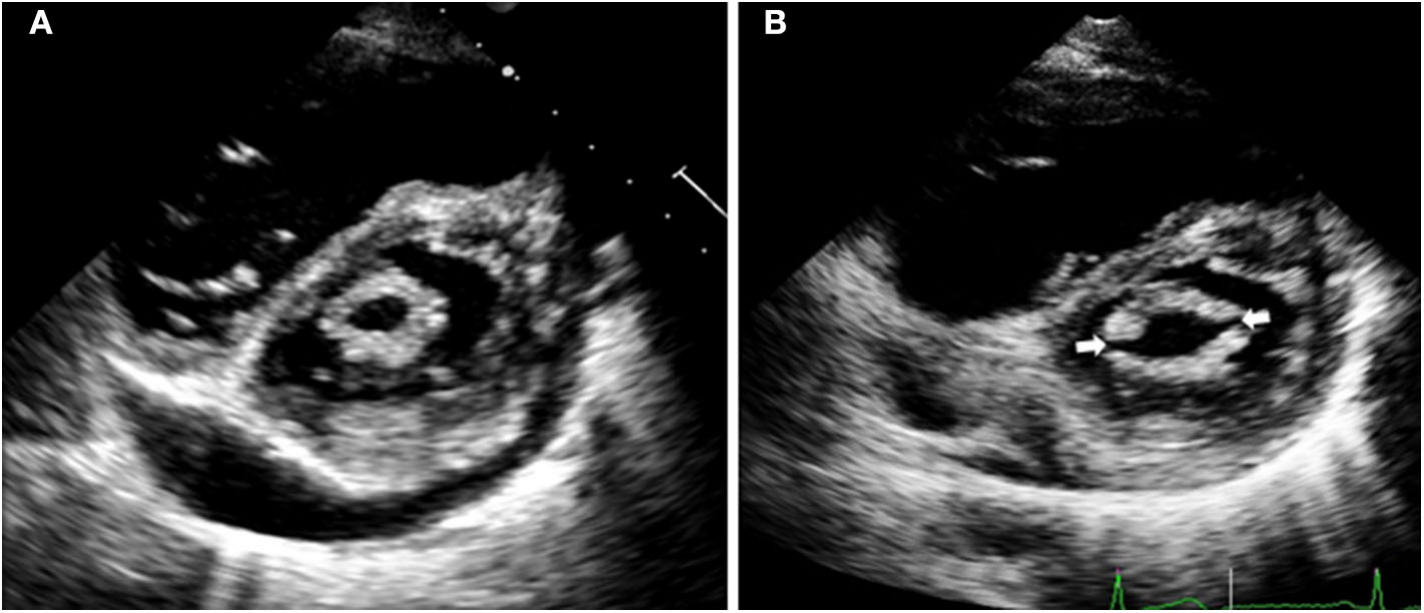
Echocardiographic images of a patient with severe mitral stenosis before percutaneous mitral commissurotomy (A). After the procedure (B), both commissures are open (arrows), which increases the mitral valve area resulting in immediate hemodynamic improvement.
